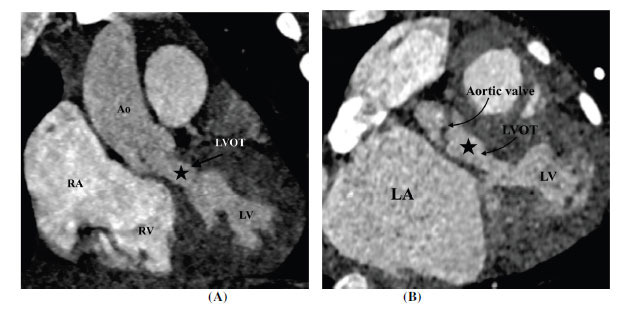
Tunnel type subaortic stenosis (A) Coronal contrast-enhanced computed tomographic angiography image and (B) oblique coronal contrast-enhanced computed tomographic angiography image showing tunnel-like subaortic stenosis (arrows). Abbreviations: RA; right atrium, RV; right ventricle, LV; left ventricle, Ao; ascending aorta.
